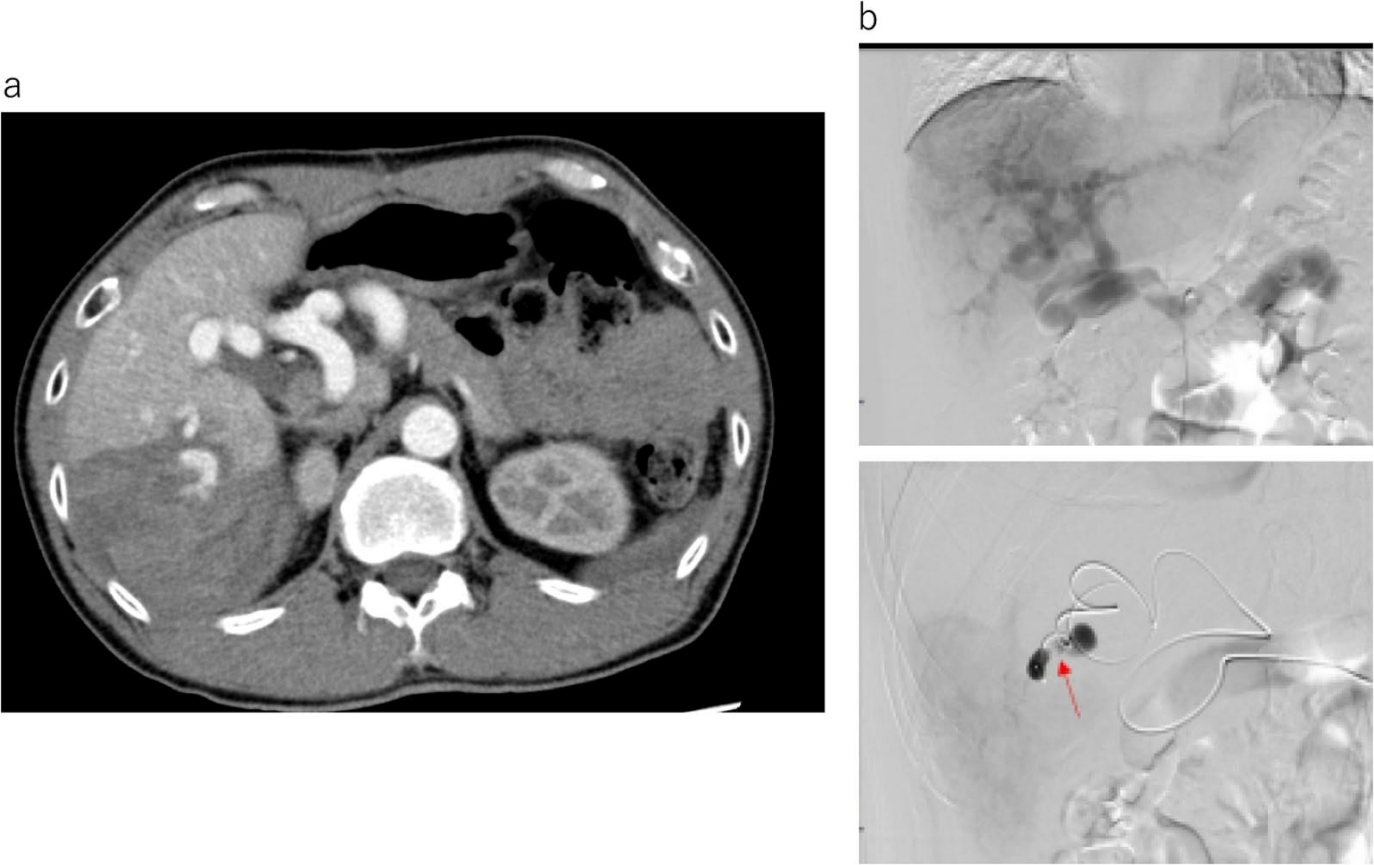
CT and interventional radiology (IVR) at abdominal hemorrhage. Enhanced-computed tomography (CT) shows poor liver regeneration of the right lobe, exposed blood vessels on the resected surface of the liver, and contrast medium leaking from the blood vessels on the resected surface of the liver into the abdominal cavity (a). An angiographic image approaching from the right external iliac artery (b). The bleeding site could not be identified by contrast imaging from the celiac artery root, because the hepatic artery was dilated and the flow velocity was fast (upper panel). When A6 was selected, the site of contrast medium leakage was confirmed (lower panel)
